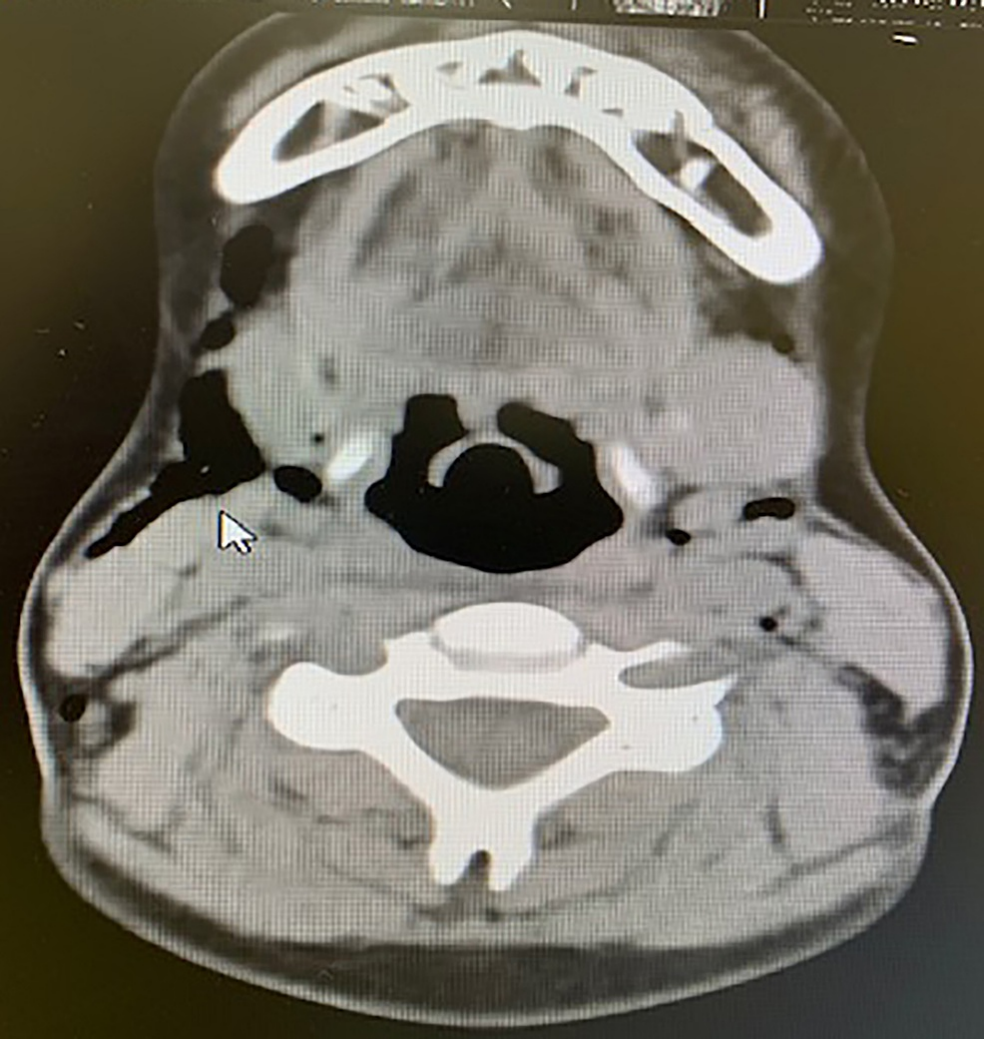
Non-contrast axial CT scan shows a significant regression of the emphysema on the fifth postoperative day.
